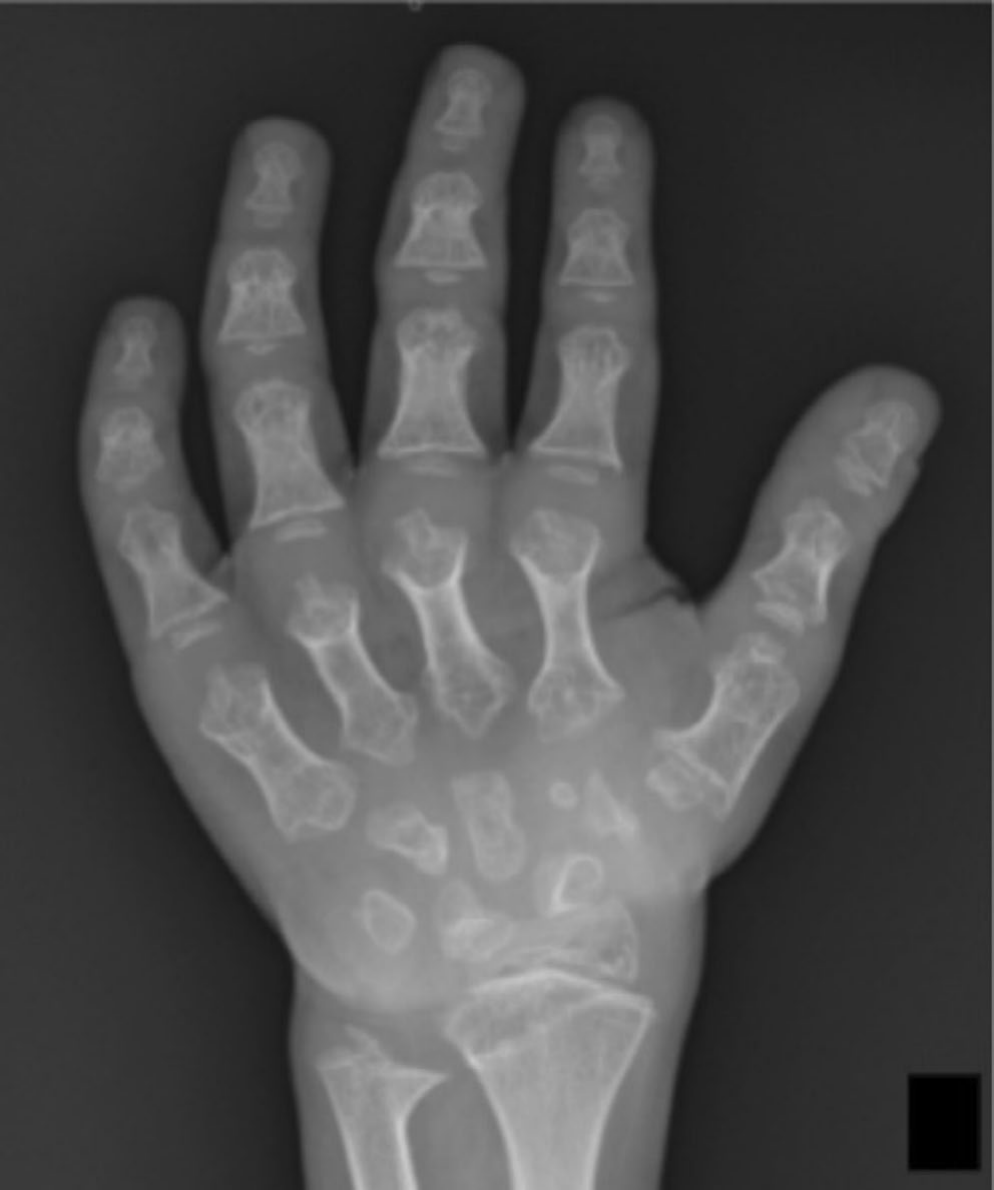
Radiograph of the left hand and wrist of a 7‐year‐old female patient with achondroplasia showing short phalanges and metacarpals, widening of the metaphyseal regions and slight separation between the middle and ring fingers are observed, characterizing the hand with a trident appearance. Negative ulnar variation.
